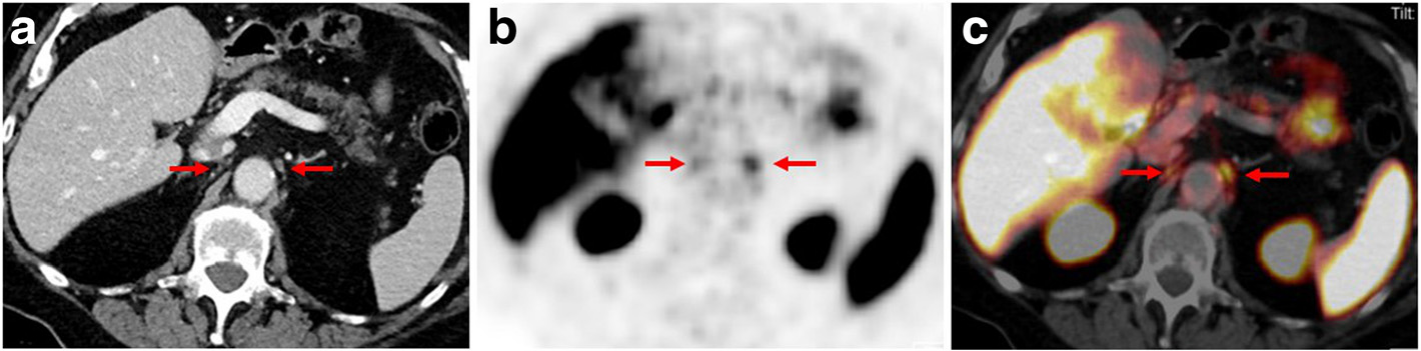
Example of a ^68^Ga-PSMA ligand PET/CT examination with incidental finding of a moderate, focal PSMA-ligand uptake in a left-sided coeliac ganglion in PET b and fused PET/CT images c. Corresponding CT images a show a teardrop-shaped soft tissue formation between the left adrenal gland and the truncus coeliacus measuring about 9 × 3 mm, typical for a benign coeliac ganglion. Additionally, faint PSMA-ligand uptake in projection on a smaller right-sided coeliac ganglion can be noted
